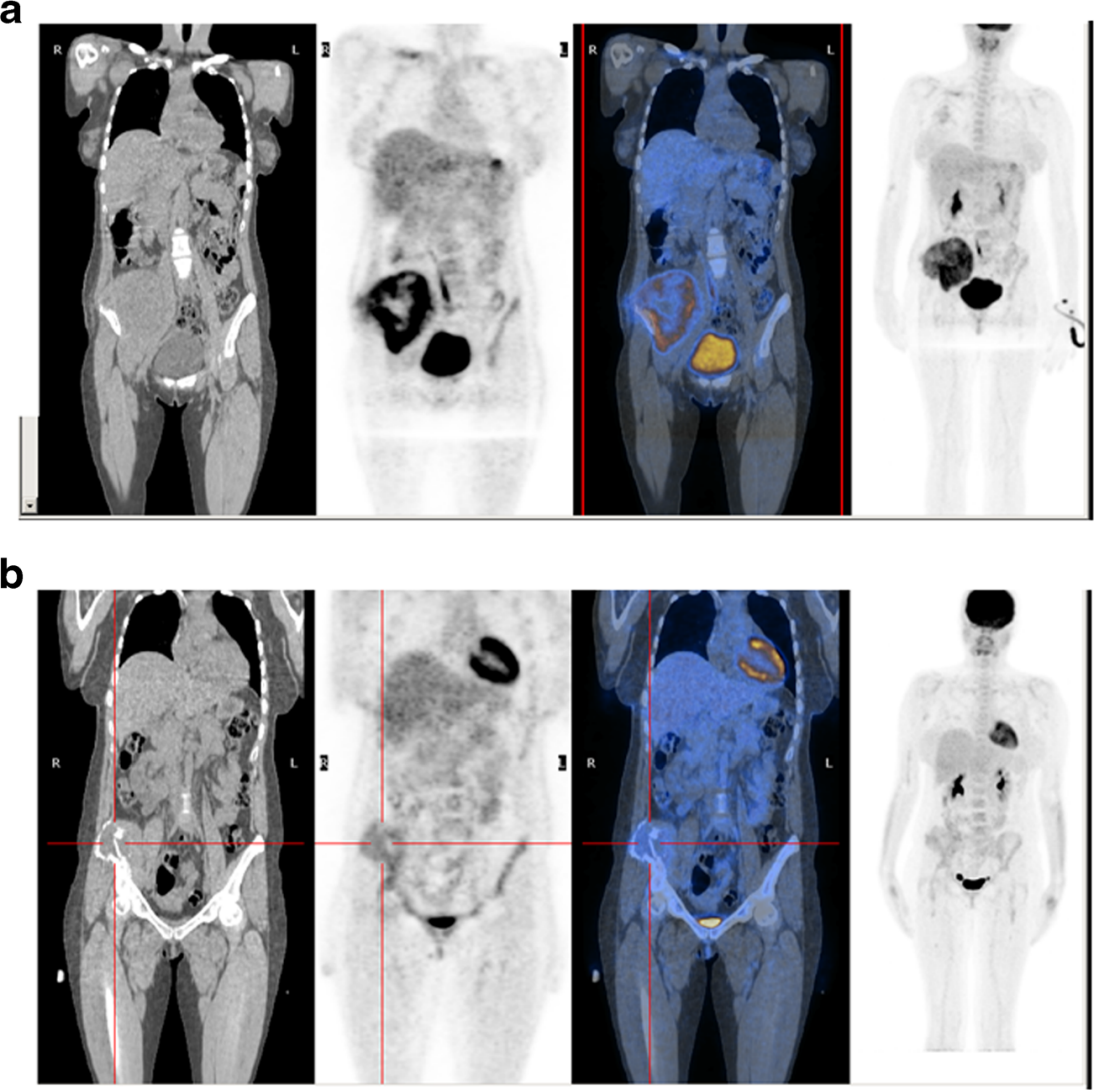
^18^F-FDG PET/CT images in a 37-year-old woman with a left iliac Ewing sarcoma before (a SUV1 10) and after (b SUV2 3.2) chemotherapy
